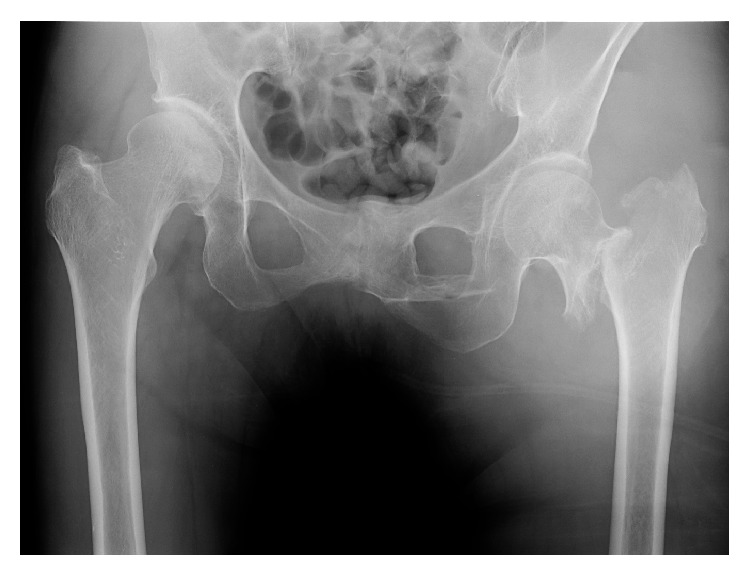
Radiograph showing a trochanteric fracture in the left femur, AO type 31-A2.2 on initial presentation.
